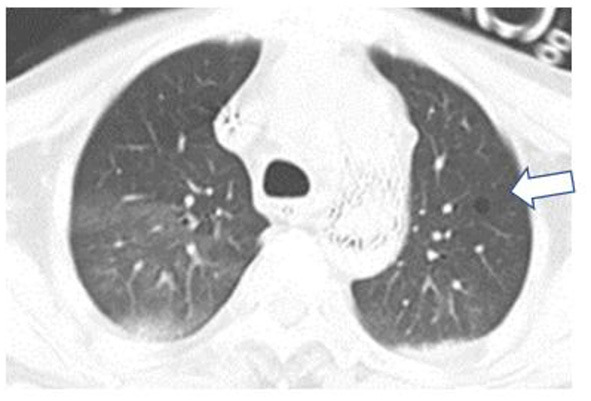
The computed tomography (CT) scans of the patient (baseline). A baseline lung CT scan noted emphysema and pulmonary bulla in the left lung. The arrow in this figure points to the pulmonary bulla of lung.
